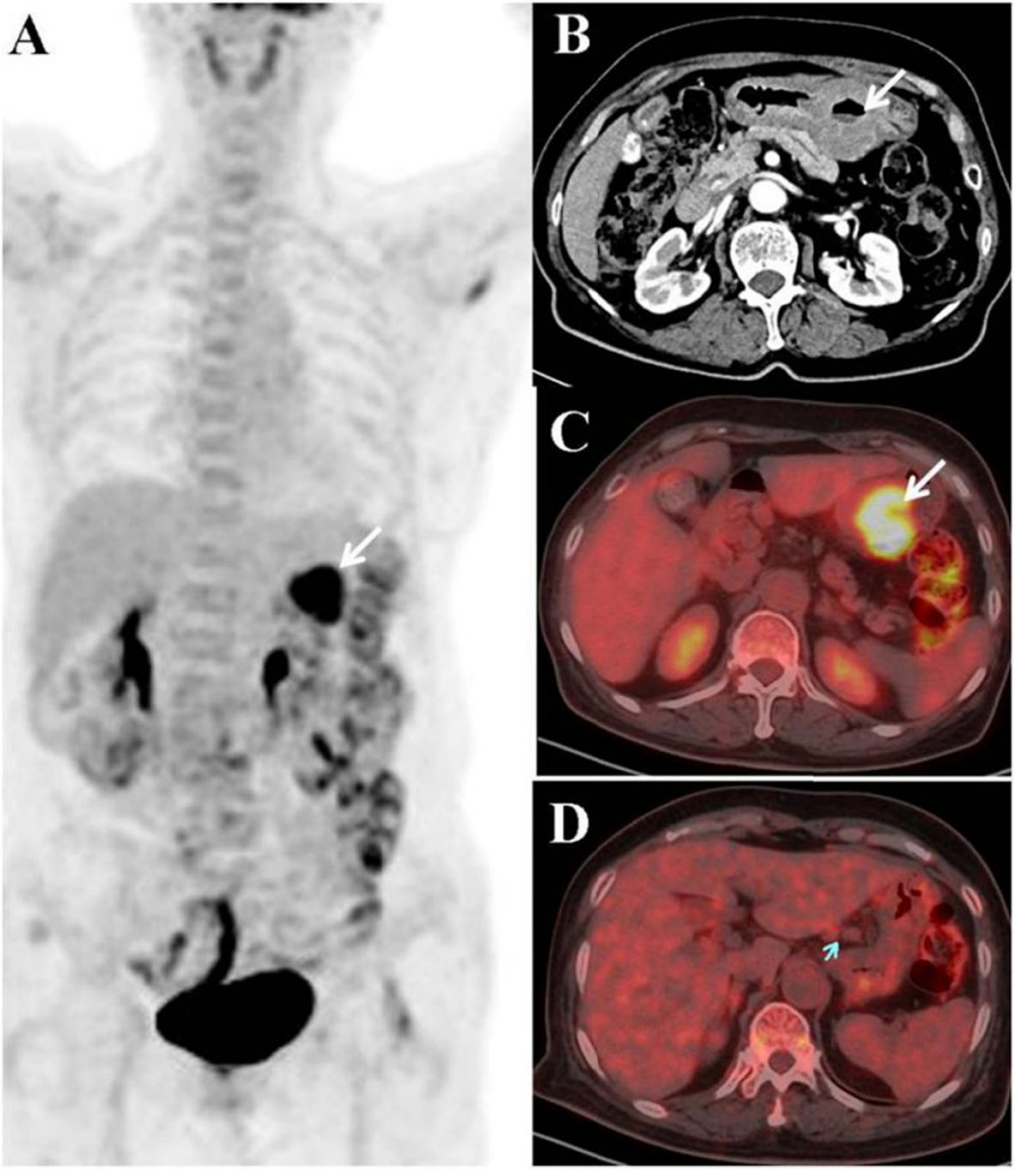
Radiographic details a^18^F-FDG PET shows a high accumulation of FDG in left upper abdomen (white arrow). b Contrast-enhanced CT view of the irregularly thickened gastric wall with slight enhancement. c Increased FDG uptake was observed in the gastric mass (SUV_max_ 14.6, white arrow). d Enlarged lymph nodes abutting the lesser curvature of the stomach, lacking FDG intensity (blue arrow)
